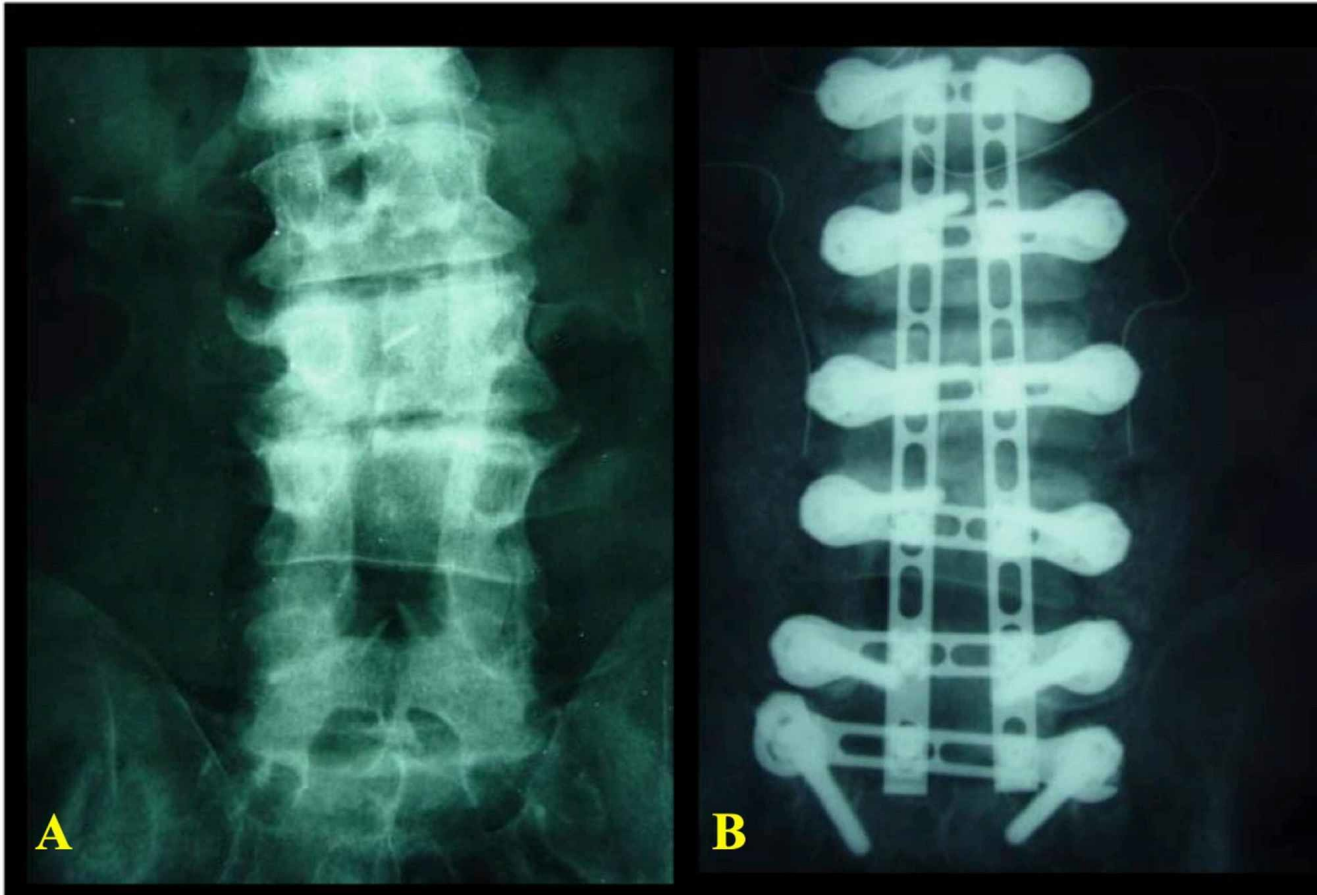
X-ray of the lumbar spine (AP view) that shows (A) levoscoliosis of the lumbar spine, (B) the lumbar spine after the correction of scoliosis with the UNIMAX system
